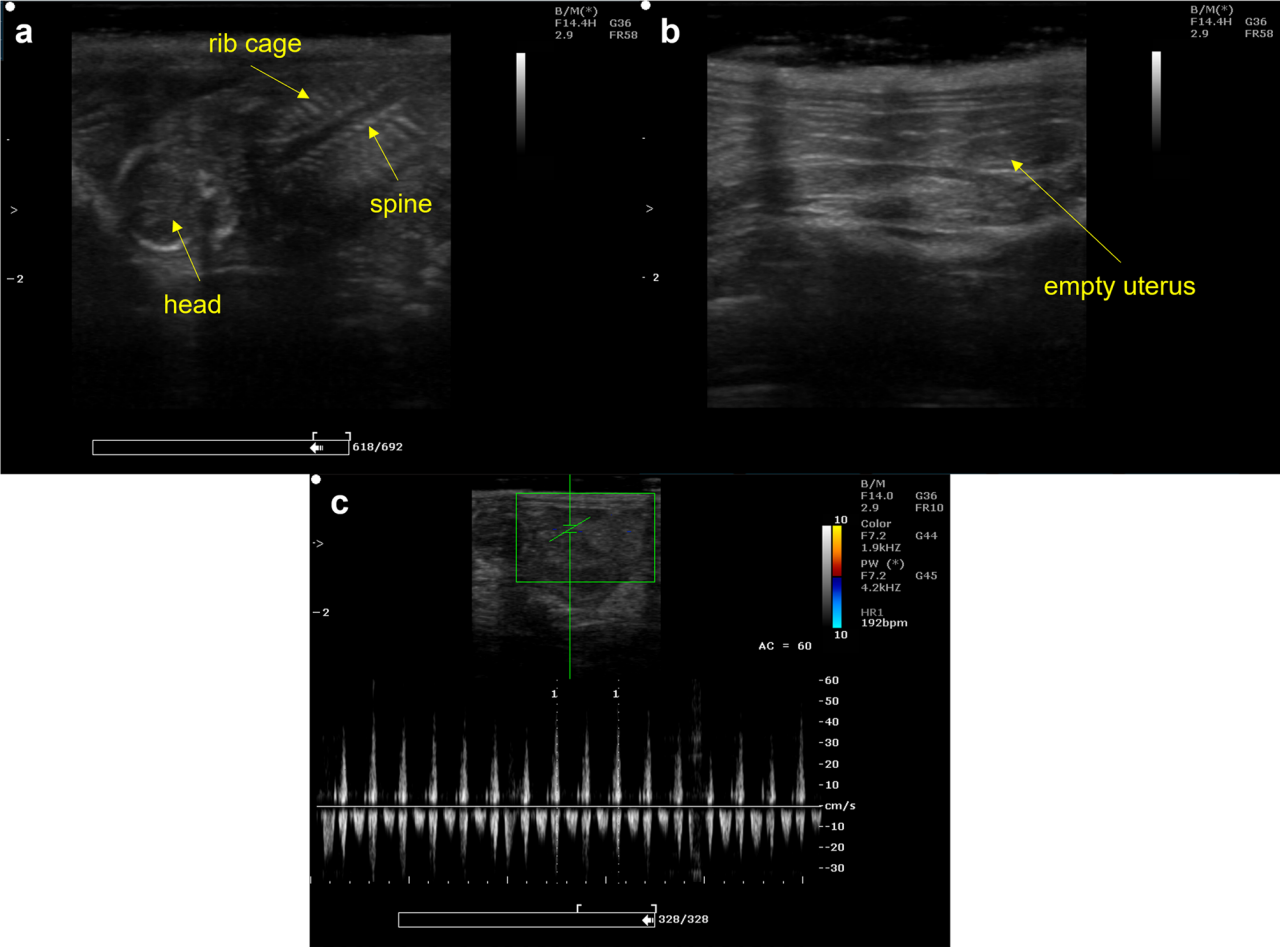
Representative ultrasound images from day 21 of gestation. (a) Sample image of a fetus with the head, spine and rib cage labelled. (b) Sample image of a non-pregnant uterus. (c) Measurement of a fetal heart rate.
